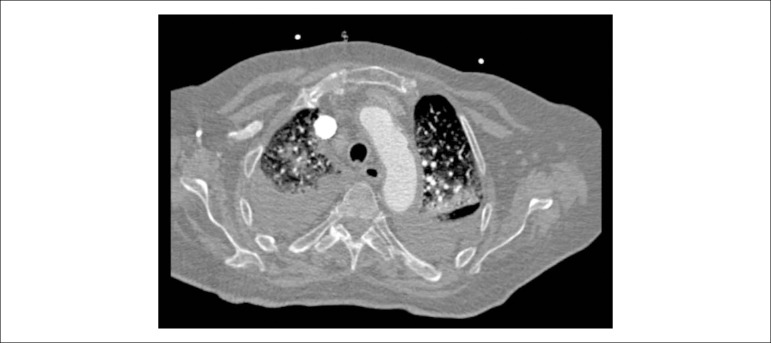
Thoracic CT scan .Link: http://publicacoes.cardiol.br/portal/abc/portugues/2020/v11402/dor-abdominal-uma-apresentacao-incomum-de-ruptura-miocardica.asp
